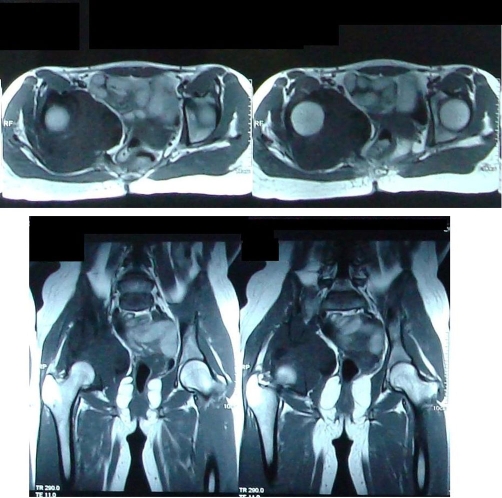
MRI pelvis of the same patient suggestive of mass lesion of right acetabulum.
